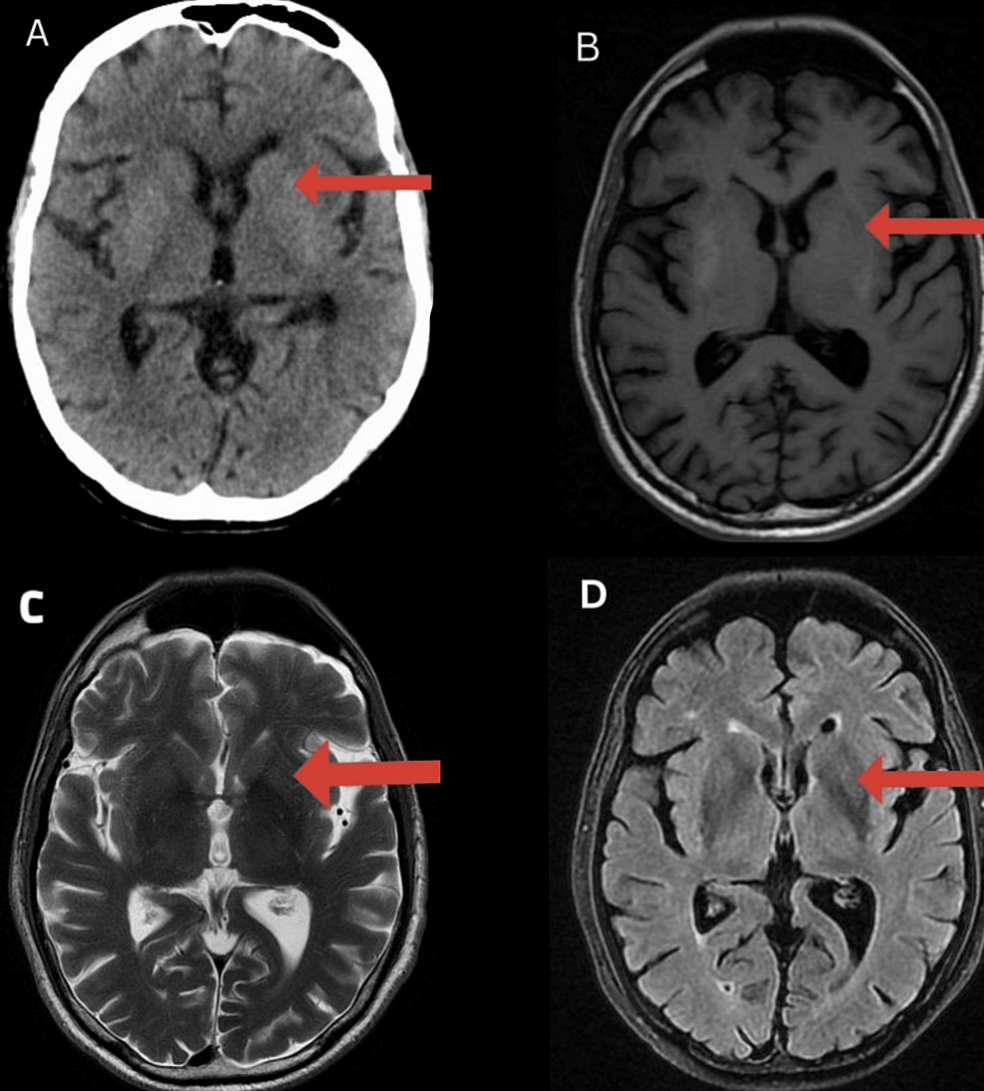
(A) Non-contrast CT scan of the brain; (B) MRI T1 sequence; (C) MRI T2 sequence; (D) MRI FLAIR sequence Arrowed areas show changes in contralateral striatum (putamen and caudate nuclei), possibly due to diabetic striatopathy. FLAIR: fluid-attenuated inversion recovery
